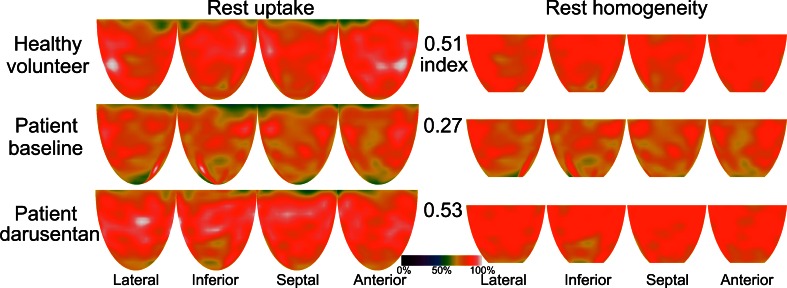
Example images. Relative uptake maps of the four LV quadrants are shown for a normal volunteer (*top row*) from our prior work^7^ as well as a notable patient at baseline (*middle row*) and while taking darusentan (*bottom row*). The *left column* shows the raw uptake images while the *right column* displays them as analyzed for homogeneity (range truncated to 50%-85%, basal four and apical two slices removed) along with the homogeneity index value. Whole LV average rest flow increased from 0.51 cc/minute/g at 7,000 bpm mm Hg at baseline to 0.74 with darusentan
